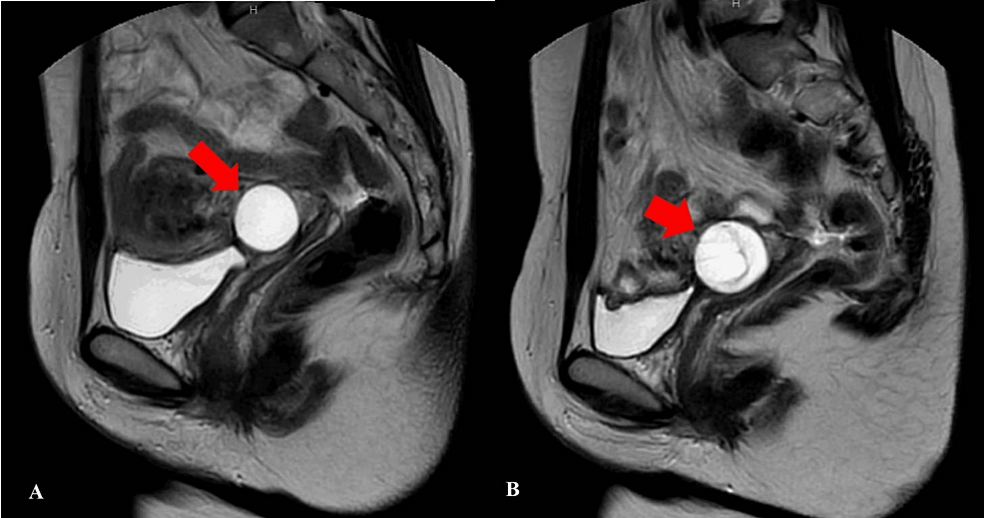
MRI images of the Case 5 patient A. MRI image at the first visit. No solid part is recognized in cervical cystic lesion; B. Second MRI just before surgery. An increase of 120% in diameter was found at 230 days in the mucinous carcinoma gastric type (GAS) case. The second MRI scan performed immediately before surgery showed no evident areas of enhancement or decreased diffusion. However, new septal structures were also observed.
